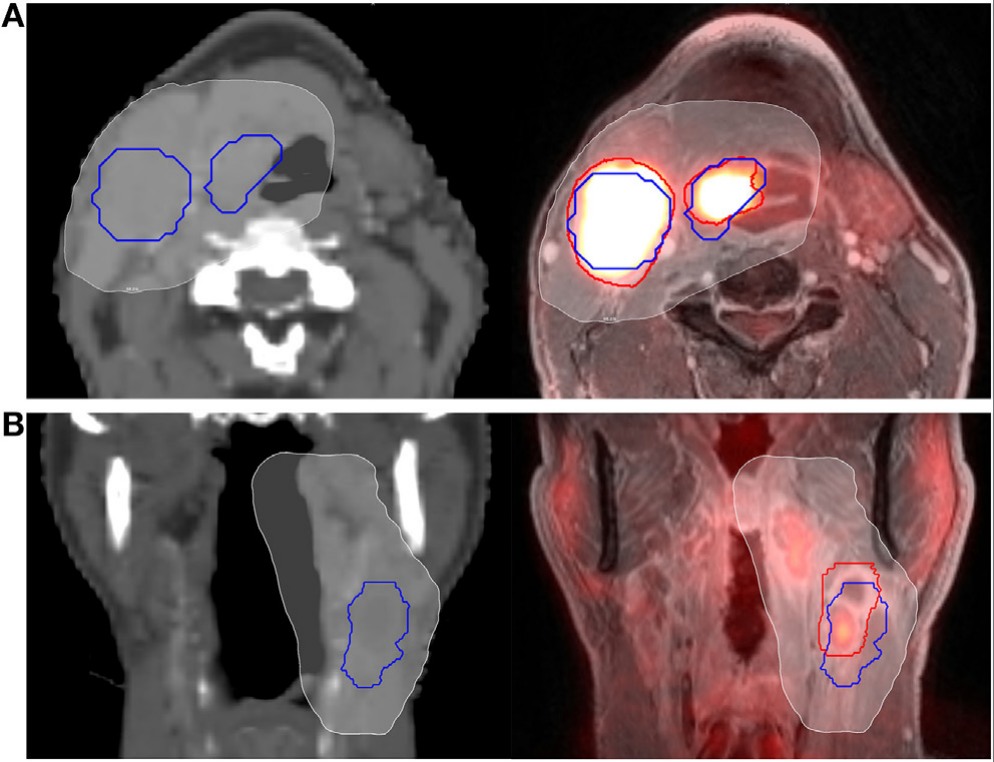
Example of computed tomography (CT) vs. PET/MRI GTV delineation. Primary tumor and nodal GTVs for two different patients (A,B) are shown delineated using CT (blue outline) and PET/MRI (red outline). The 95% prescription isodose line from their CT-based treatment plan is overlayed (white, shaded). The patient in panel (A) (axial representation) had fair spatial overlap between PET/MRI and CT volumes, with a Dice similarity coefficient (DSC) of 0.72 for the primary GTV and 0.69 for the nodal GTV. The patient in panel (B) (coronal representation) had relatively poor spatial overlap between the PET/MRI and CT nodal volumes, with a DSC of only 0.48. Clinical target volume and planning target volume expansions are not shown.
